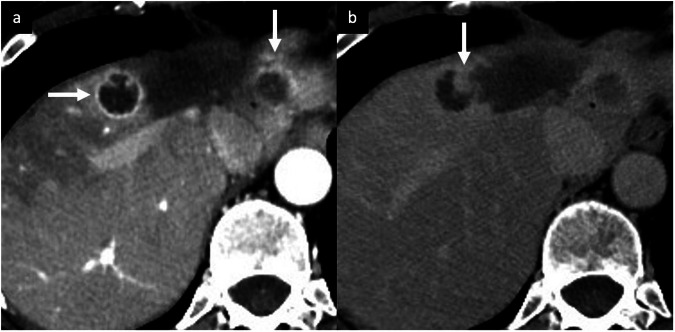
Multinodular tumor recurrence of iCCA in a 49-year-old woman. CT performed after local-regional treatment (transarterial radioembolization) demonstrates multinodular tumor recurrence around the treated zone showing irregular, thickened, nodular rim APHE (a, arrows) which persisted and increased (b) on DP (arrow)
